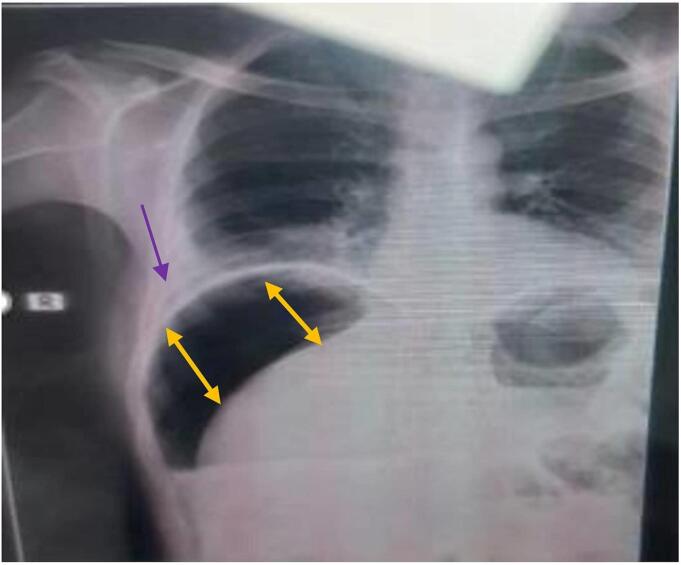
Chest X-ray at hospital admission: Shows free air under the diaphragm (double yellow arrows), obliterated right costo-diaphragmatic angle (purple arrow). (For interpretation of the references to colour in this figure legend, the reader is referred to the web version of this article.)
